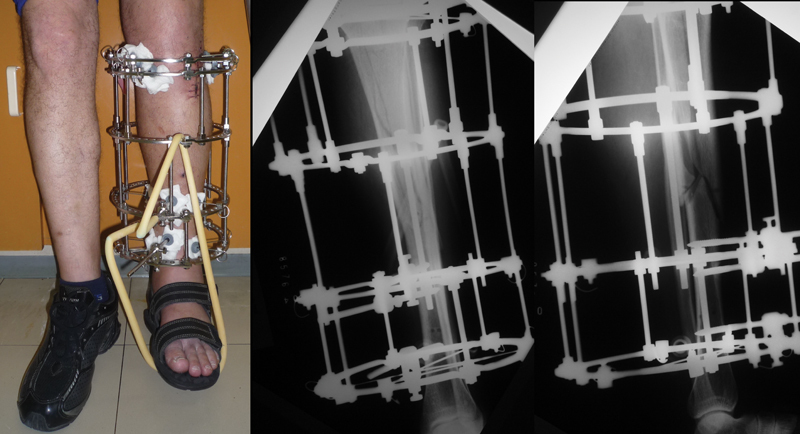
Clinical and radiographic images demonstrating the classic assembly in a patient included in the study.
